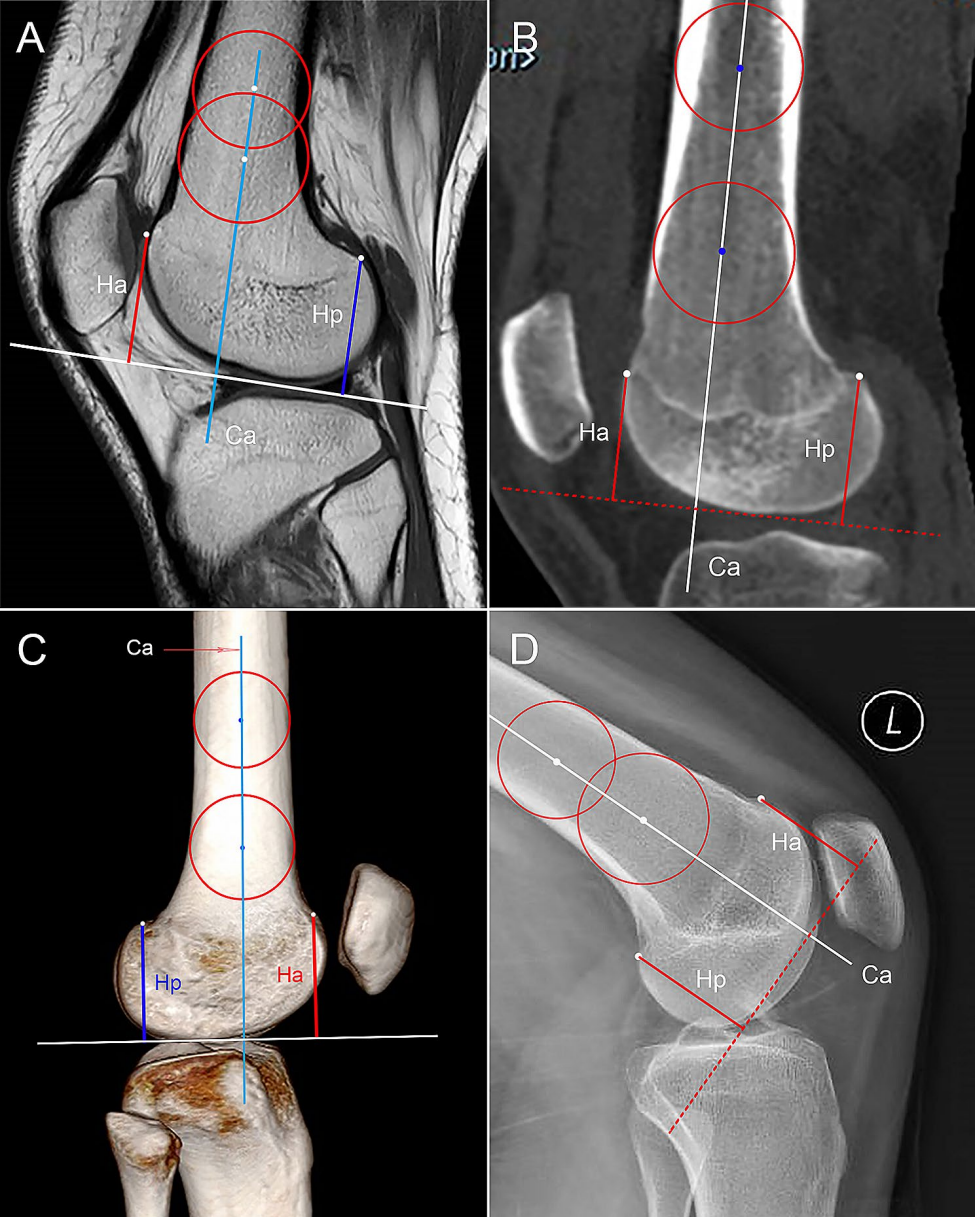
The measurement of lateral condyle index (LCI). (A) Midsagittal MRI slice is selected. Two circles tangent with the cortex of distal femur are drawn to identify the central longitudinal axis (Ca). The highest portion of anterior and posterior femoral condyle cartilage (white dots) are marked to measure to height of femoral condyles (Ha and Hp, respectively). LCI is defined as the ratio of Hp/Ha. Similarly, referring to the continuous portions of femur cortex and femoral condyle (white dots), the LCI is measured on sagittal CT slice (B), on 3D–CT (C), and on conventional radiograph (D) by similar methods
